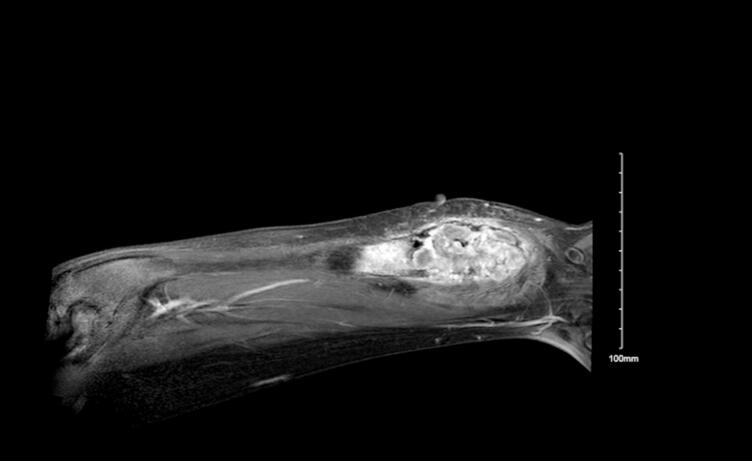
Magnetic resonance image obtained 1 year after the initial presentation. Coronal image demonstrating an expansive lesion involving the distal third of the left ulna with evidence of fluid-fluid level.
